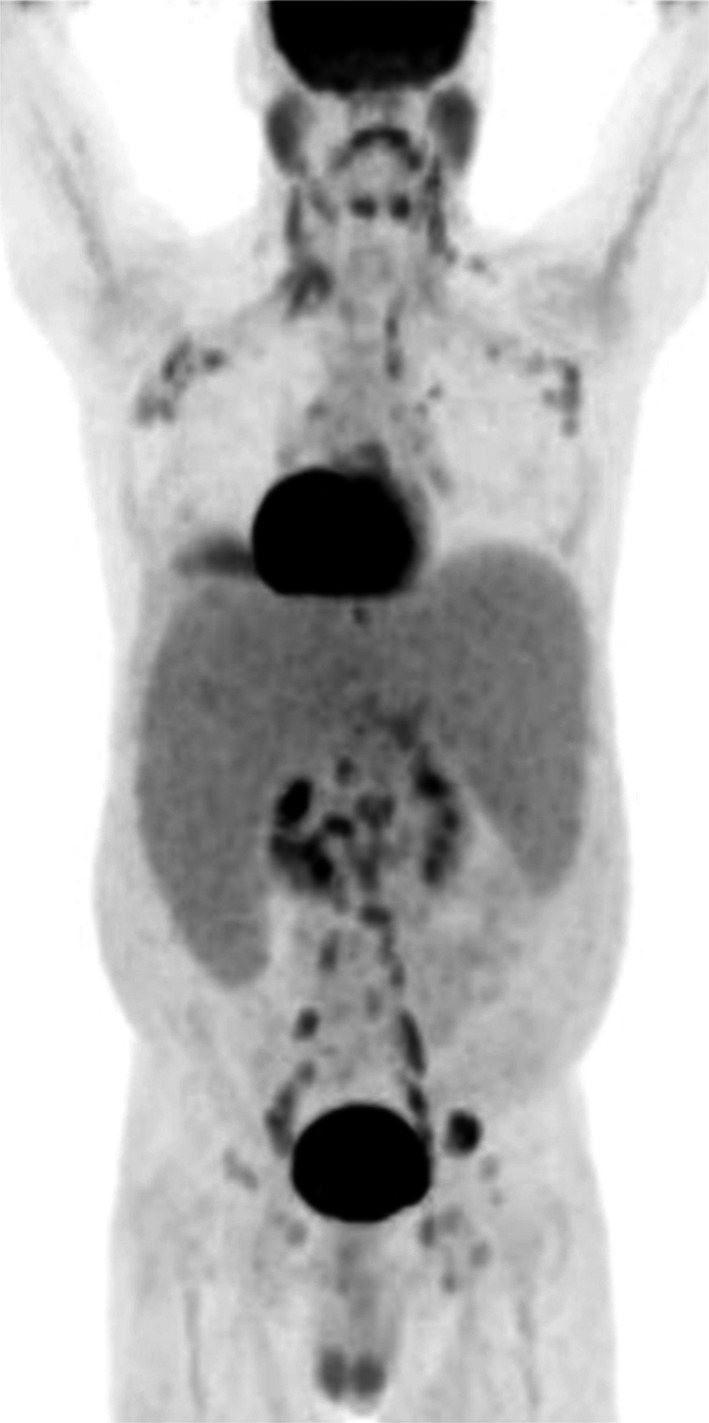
A PET/CT scan showing spleen length of 31 cm, liver length of 26 cm, and diffuse bulky lymphadenopathy
